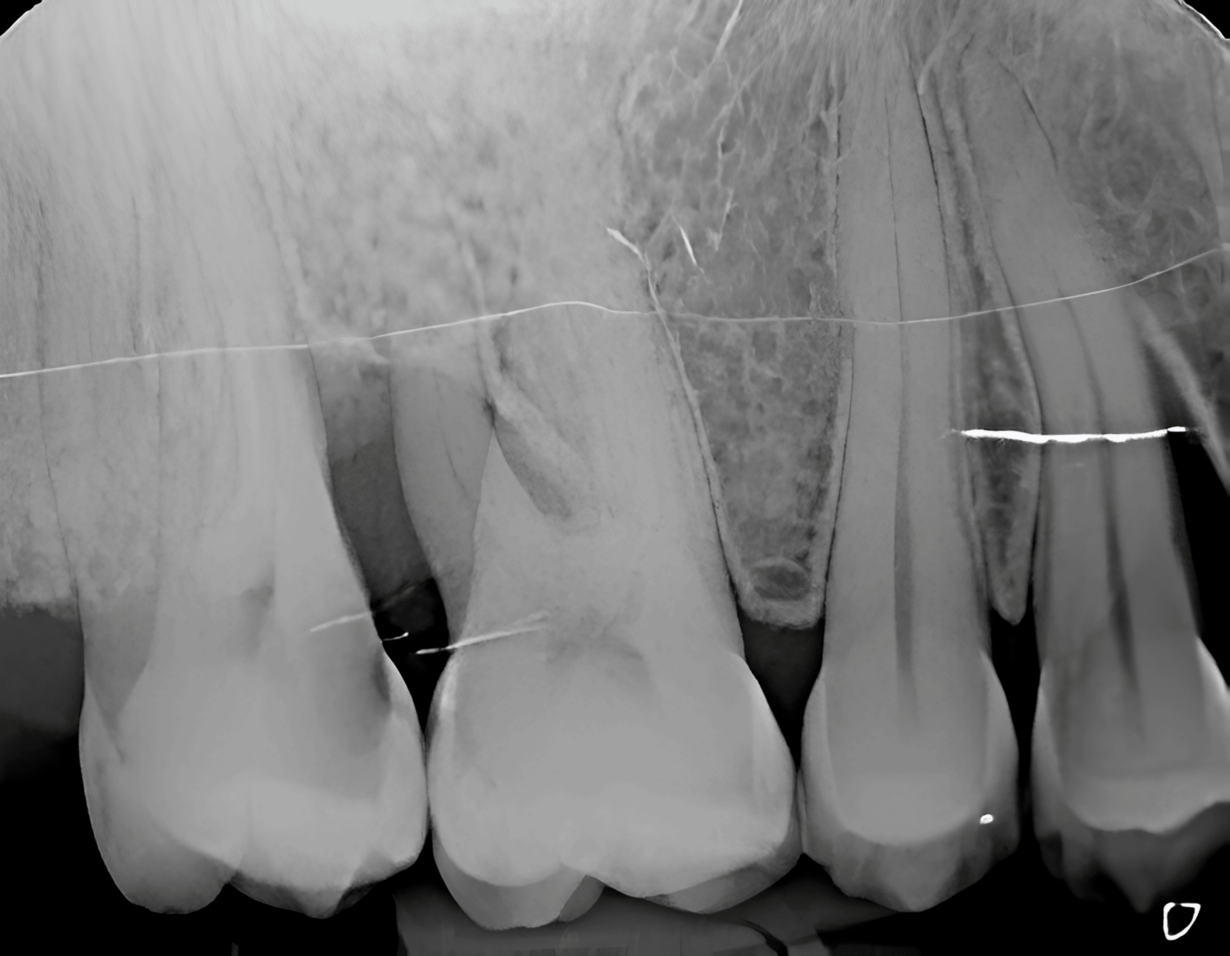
Periapical radiograph of teeth #15 and #16
